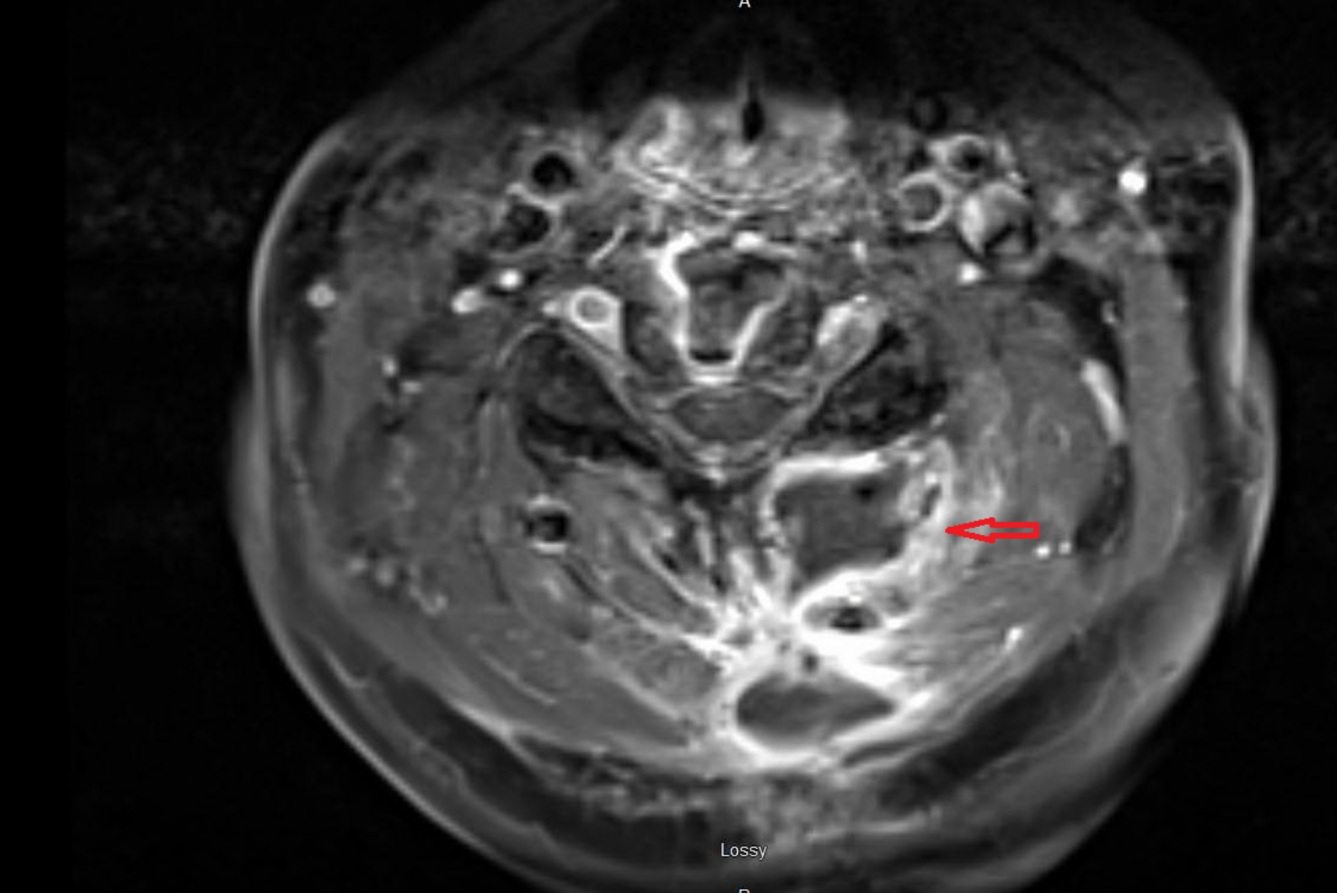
Axial MRI postsurgical resection. T1 weighted enhanced MRI of the cervical spine four weeks after resection, showing gross total resection with associated postoperative changes in the axial plane.
